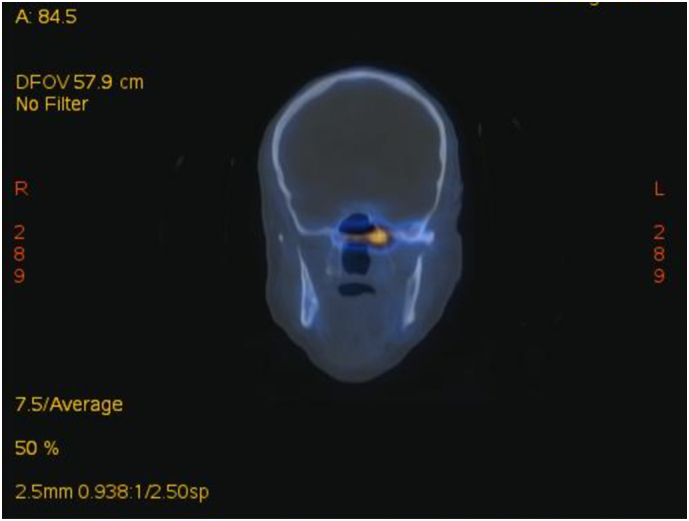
Bone scan showing scintigraphic findings are suggestive of bone remodeling of left middle ear bones extending to mastoid air cells and sphenoid bone suggestive of active bone infection.
